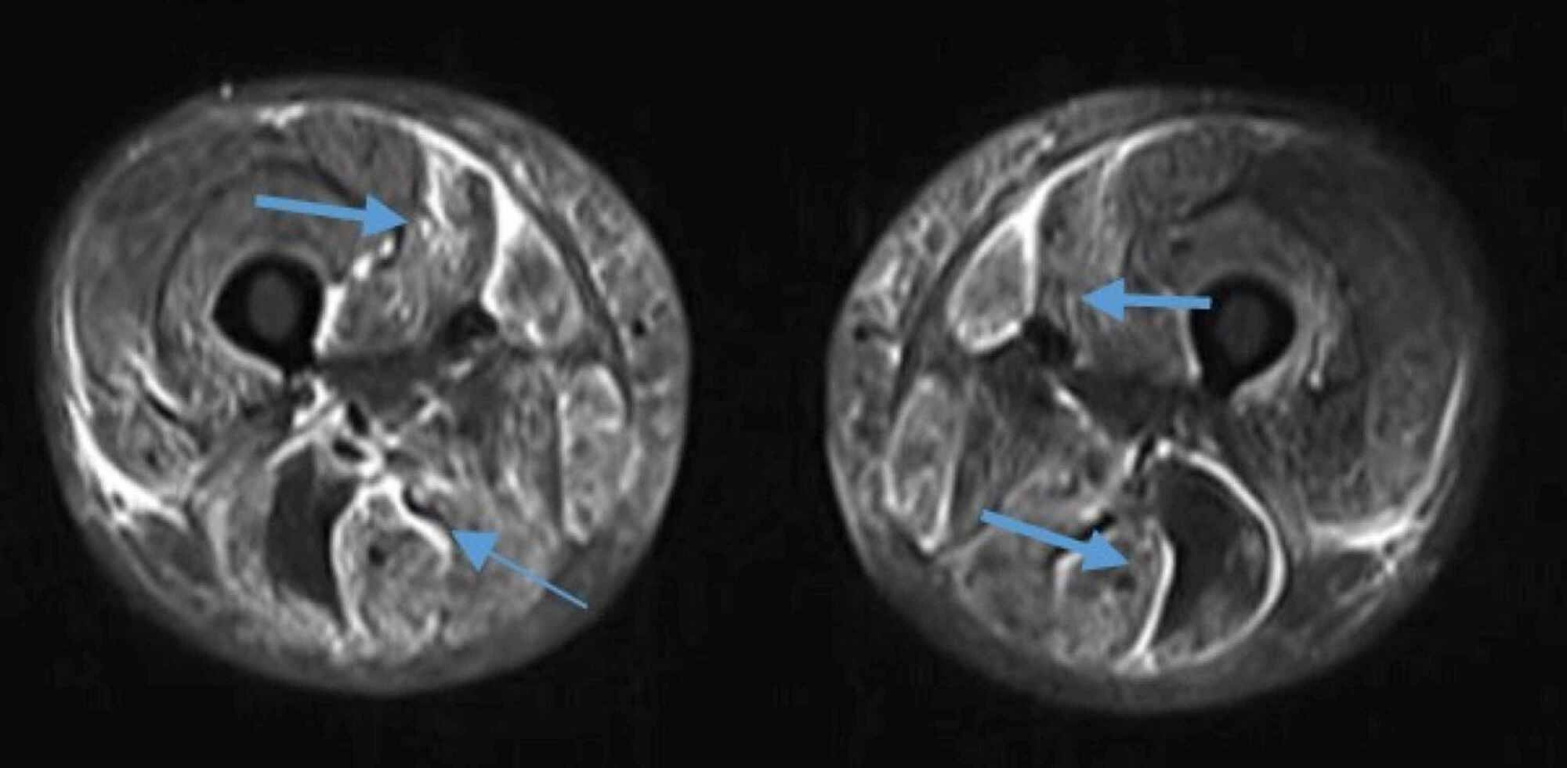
T2-weighted MRI thighs showing altered signals in thigh muscles (predominantly in adductors and extensors) symmetrically with circumferential involvement.
